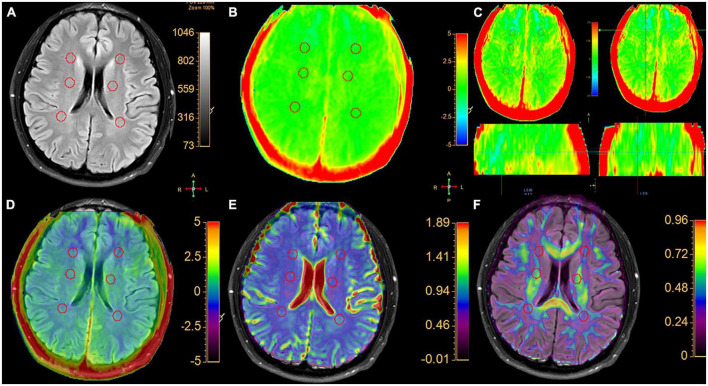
A typical healthy control. (A) Six spherical VOIs with a volume of 0.5 cm^3^ were placed bilaterally on normal white matter in the frontal white matter, centrum semiovale and parietal white matter, respectively. (B) APTw image with a –5 to 5% display range. (C) APTw images of three planes with a –2.5 to 2.5% display range. In three planes, the signal distribution of MTRasym (3.5 ppm) is uniform. (D) APT and FLAIR image fusion. (E) ADC and FLAIR image fusion. (F) FA and FLAIR image fusion.
